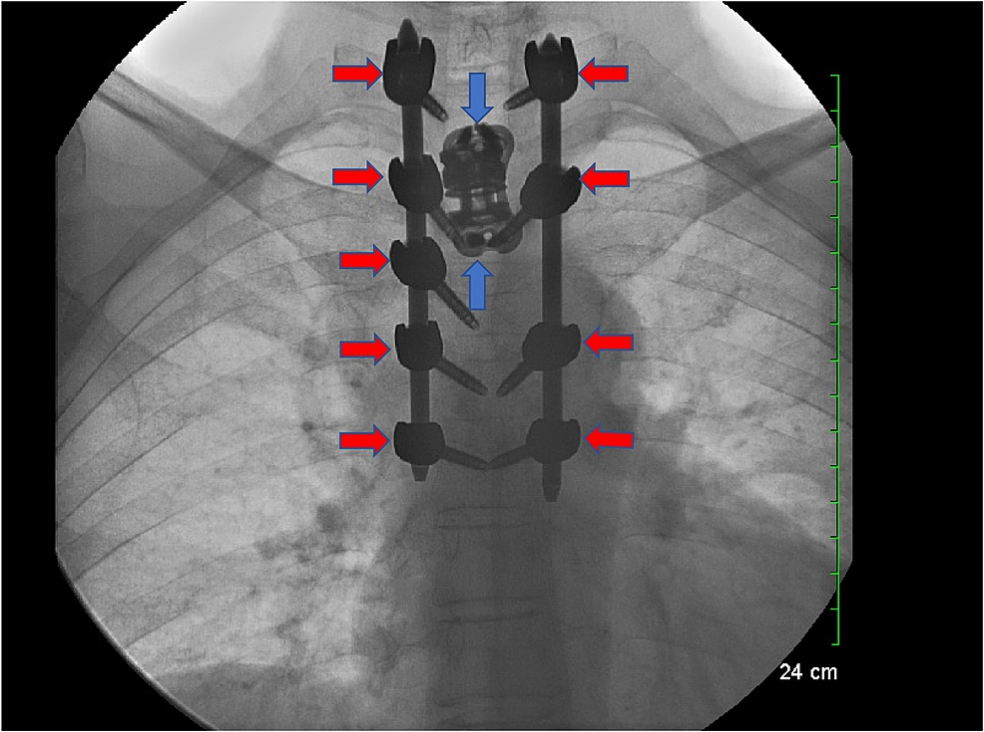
Postoperative anterior-posterior XR of the thoracic spine showing proper positioning of the wires (red arrows) and interbody cage (blue arrows) XR: x-ray
